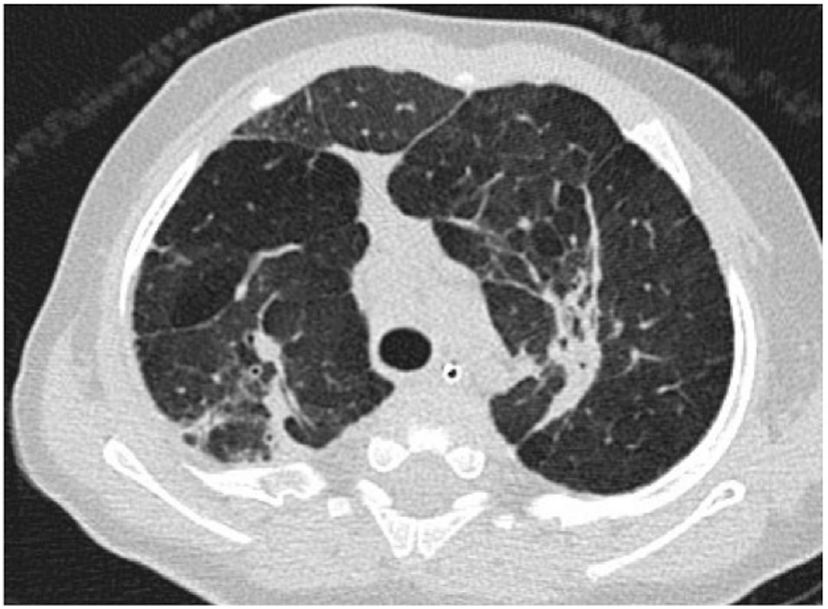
Axial computed tomography section through the upper lobes on lung window
